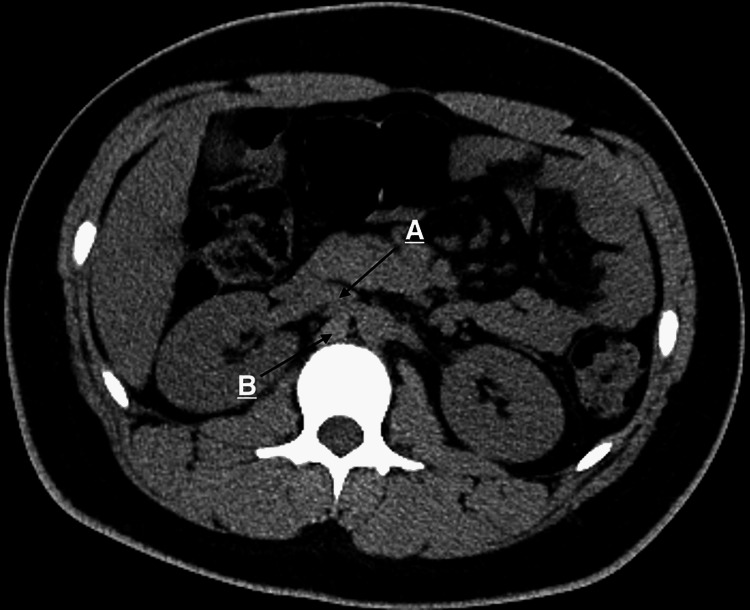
An axial CT scan. A - The right renal artery. B - A right hypertrophied diaphragmatic crus indenting the proximal right renal artery.
